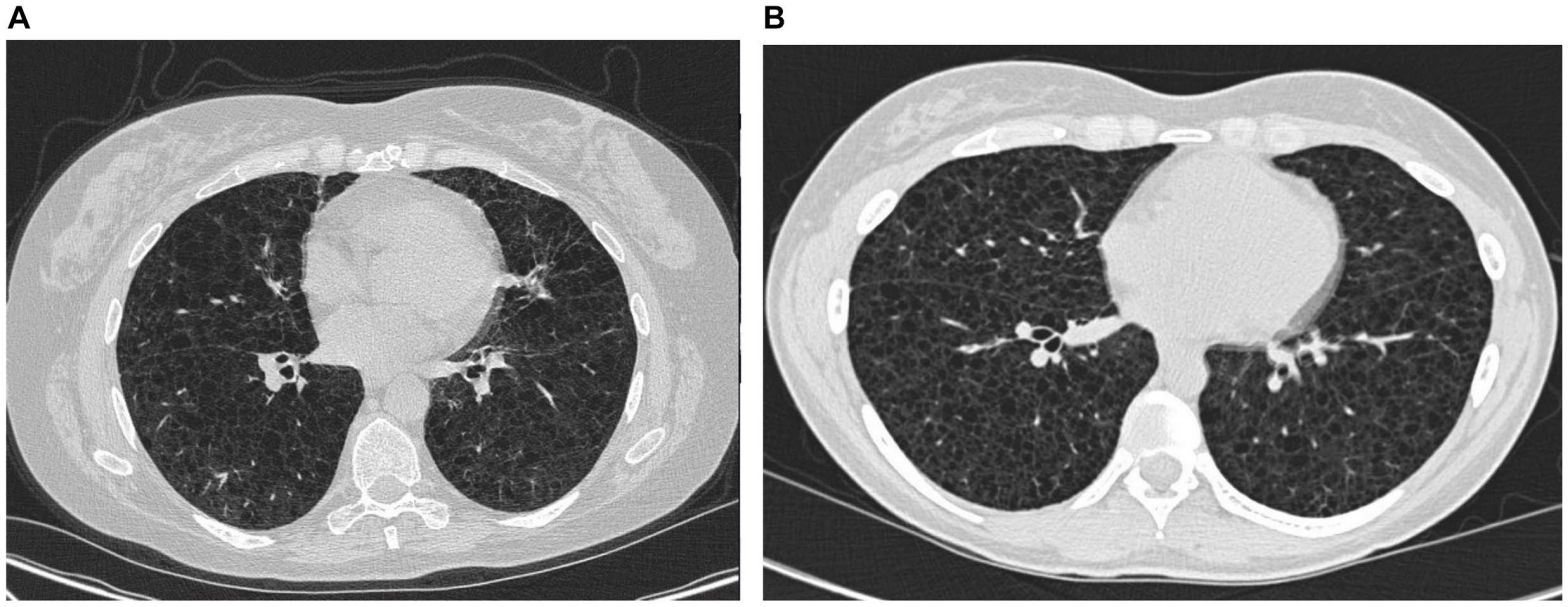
High-resolution computed tomography from case 1 (A) and case 2 (B).
